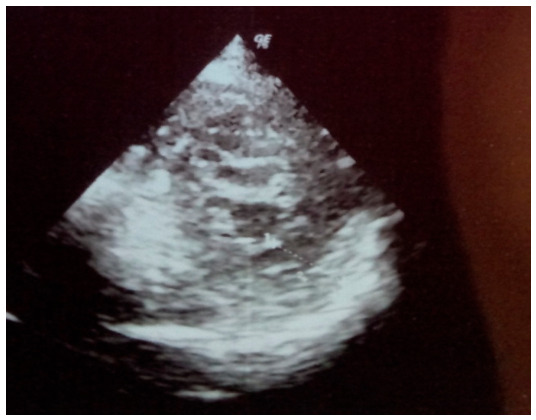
Cardiac ultrasound appearance of LVNC, with trabeculates.
